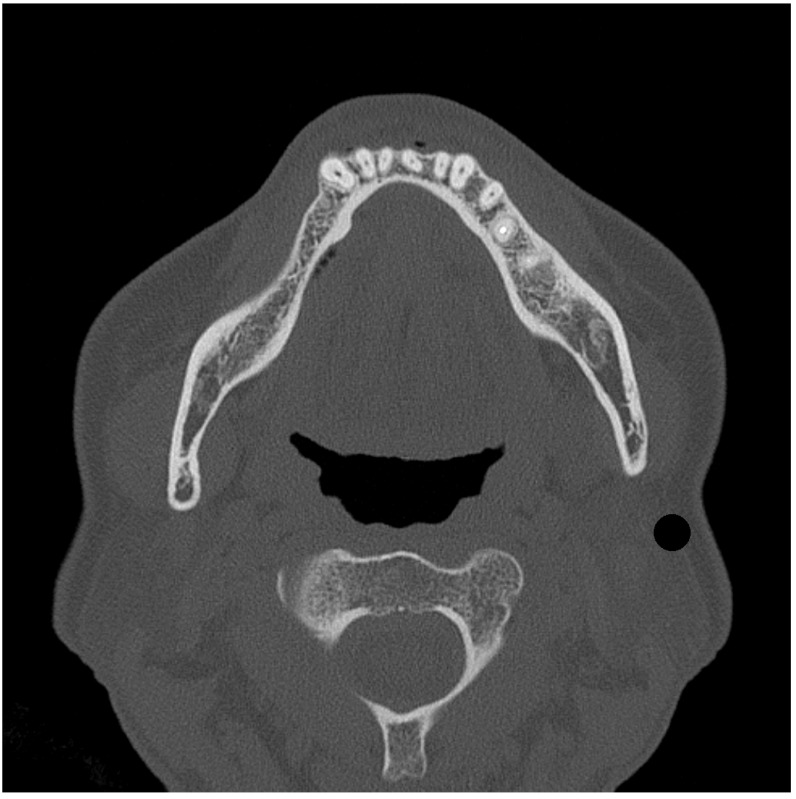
CT data (DICOM) for mandibular bone.
